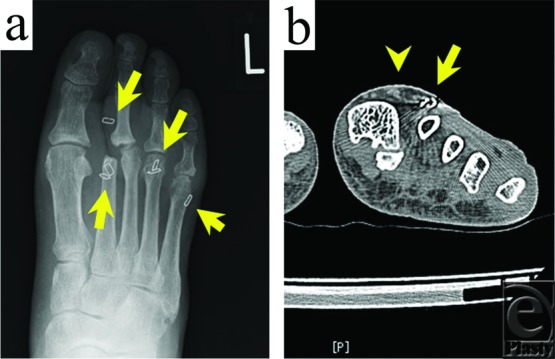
Radiograph and computed tomographic scan. (a) Radiograph showing the presence of skin staples (arrow) at the amputation stump. (b) Computed tomographic scan showing the lesion (arrowhead) overlaying a residual skin staple (arrow); no tumor invasion to the surrounding tissue was evident.
